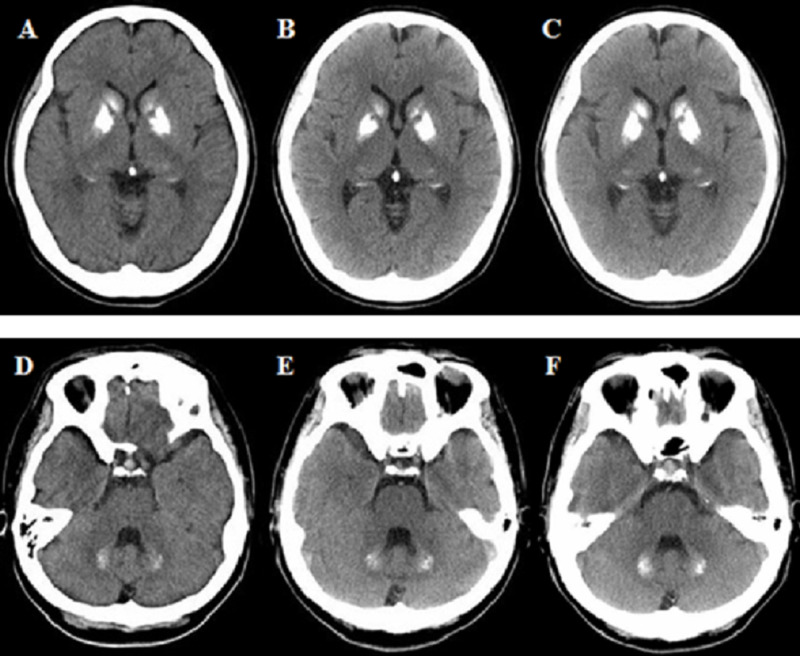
Longitudinal follow-up of the brain calcification in cranial CT Basal ganglia at the age of 37 years (A), 43 years (B), and 47 years (C); cerebellar dentate nuclei at the age of 37 years (D), 43 years (E), and 47 years (F).
